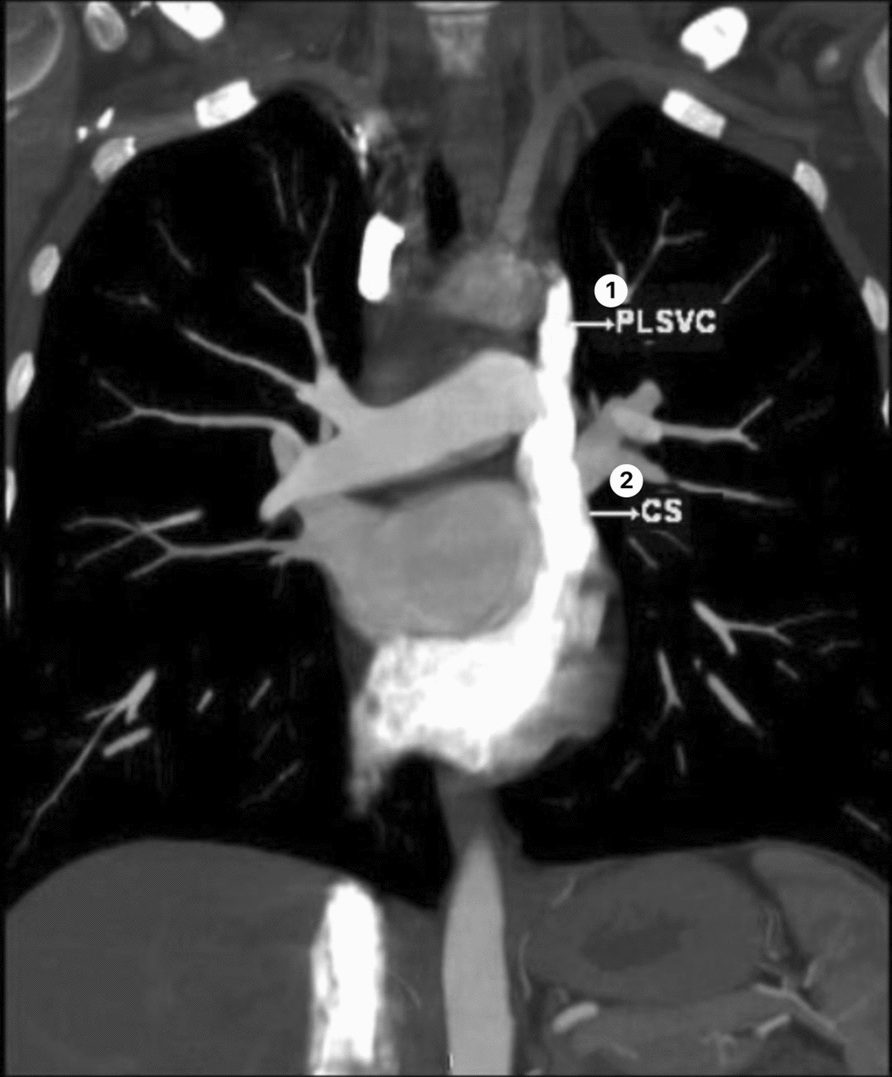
This image shows contrast-enhanced computed tomography revealing a persistent left superior vena cava draining into a dilated coronary sinus. (1) PLSVC, persistent left superior vena cava; (2) CS, coronary sinus
